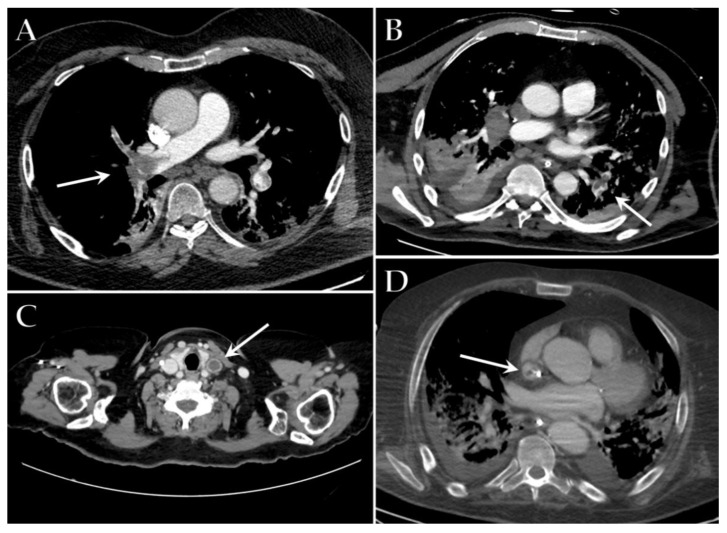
Axial contrast-enhanced CT images of different ICU patients with COVID-19 show filling defects indicative of a massive lung embolism in the right pulmonary artery (white arrow) (A), multiple embolisms in the left lower lobe artery (white arrow) (B), thrombosis of the left jugular vein (white arrow) (C) and thrombosis of the superior vena cava near the central venous catheter (white arrow) (D).
